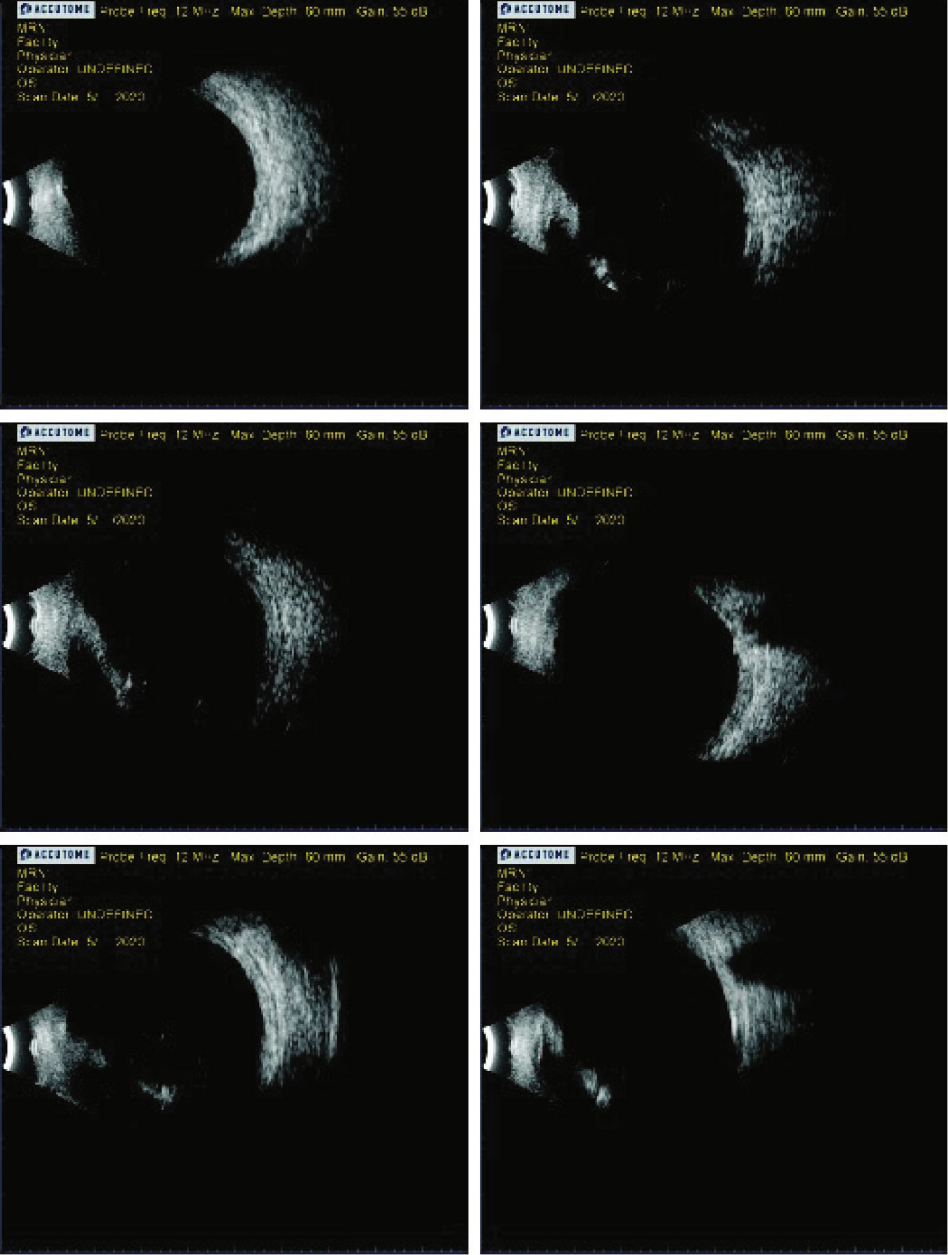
Ultrasound B-scan from postop day 90 (following vitrectomy). Mild vitreous haziness and visual artifacts present but no choroidals.
